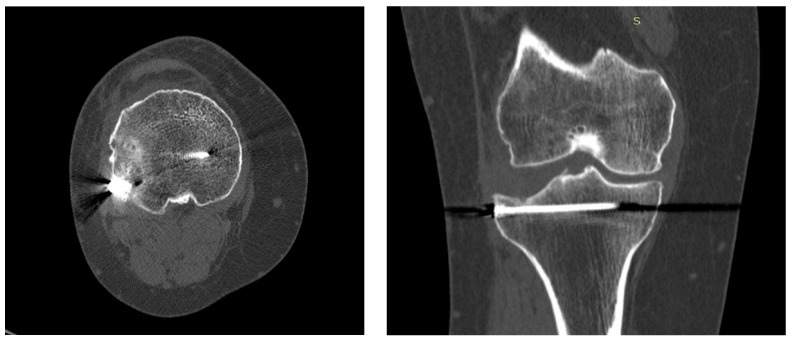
Axial (left) and coronal (right) computed tomography of the right knee, at 18 months follow-up.
